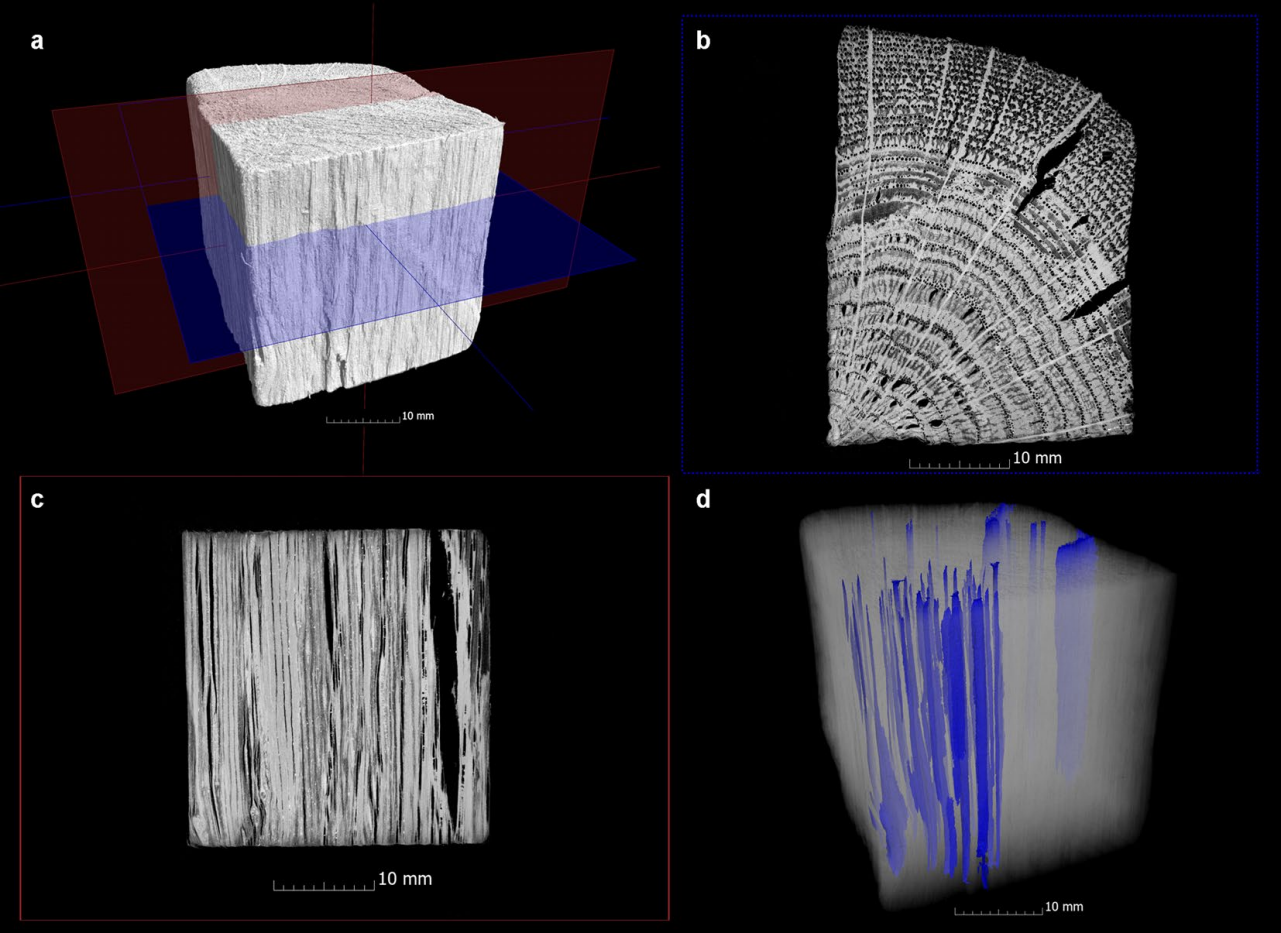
CT visualisation of the collapse in an oak sample (Oa-Sac-3) conserved with Saccharose. The position of the cross section (b) and longitudinal section (c) are shown as blue and red planes in the 3D visualization (a). The 3D visualization (d) shows the collapse in blue colour. While in better preserved wood the collapse is smaller and limited to annual rings but occurs more frequently, in poorly preserved wood there are fewer but larger areas that have collapsed.
